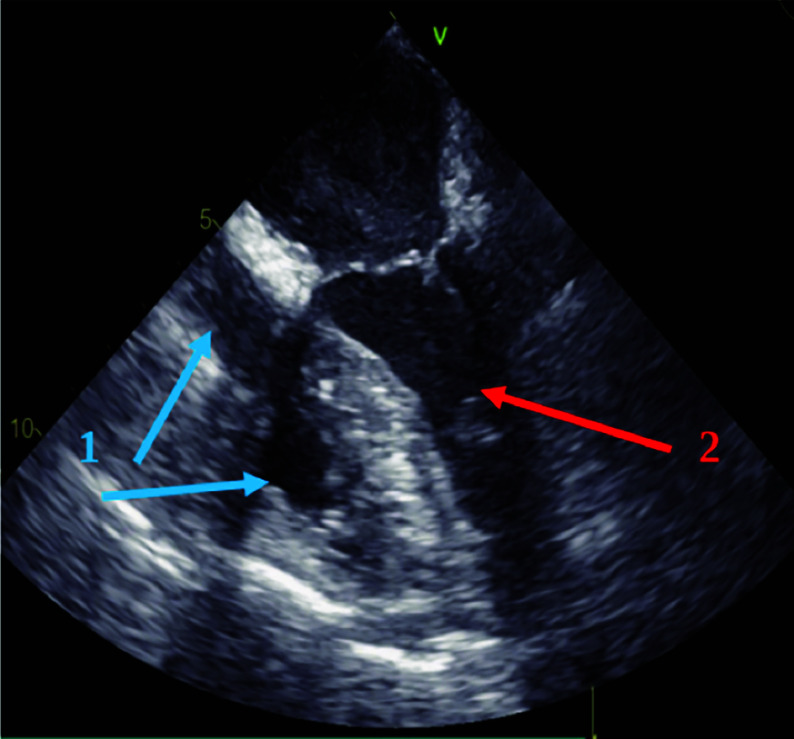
Transesophageal echocardiography picture. The right chambers of heart are collapsed due to snared superior and inferior cava veins (1, blue arrows); reduced end diastolic volume of left ventricle (2, red arrow).
